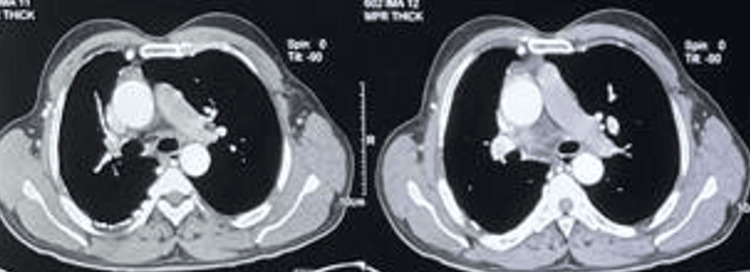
Mediastinal section thoracic CT scan: the absence of the right pulmonary artery and left-sided dominance of pulmonary blood flow
